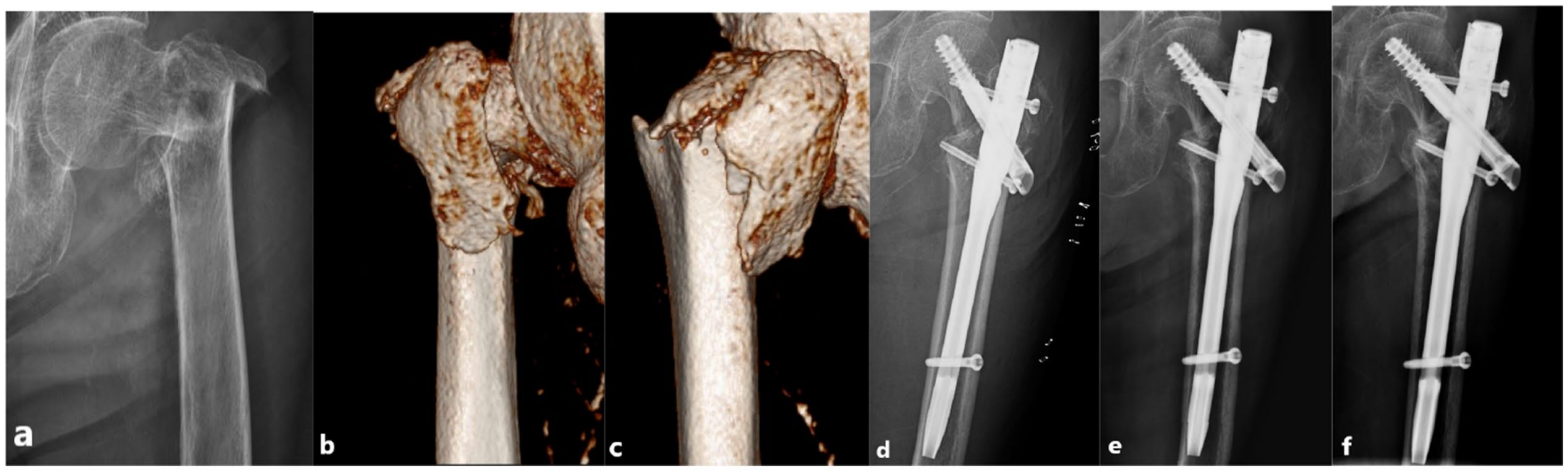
PFUN internal fixation in a 79-year-old female patient with a left intertrochanteric fracture. The patient experienced pain in the left hip and limited movement after the fall, and was unable to walk. At the last follow-up at 16 months after the operation, the Harris score was 89 points and the patient can walk independently. (a) X-ray image showing intertrochanteric fracture of the femur after the injury. (b) CT showing the fractures of the medial femoral wall. (c) CT showing the fractures of the lateral femoral wall. (d) X-ray image immediately after the operation showing appropriate fracture reduction. The lesser trochanteric fragment (the medial femoral wall fragment) was fixed with a lesser trochanteric screw and the lateral femoral wall fragment was fixed with a lateral wall screw. (e) X-ray image showing appropriate internal fixation evolution 3 months after the operation. (f) X-ray image showing appropriate fracture healing 12 months after the operation.
